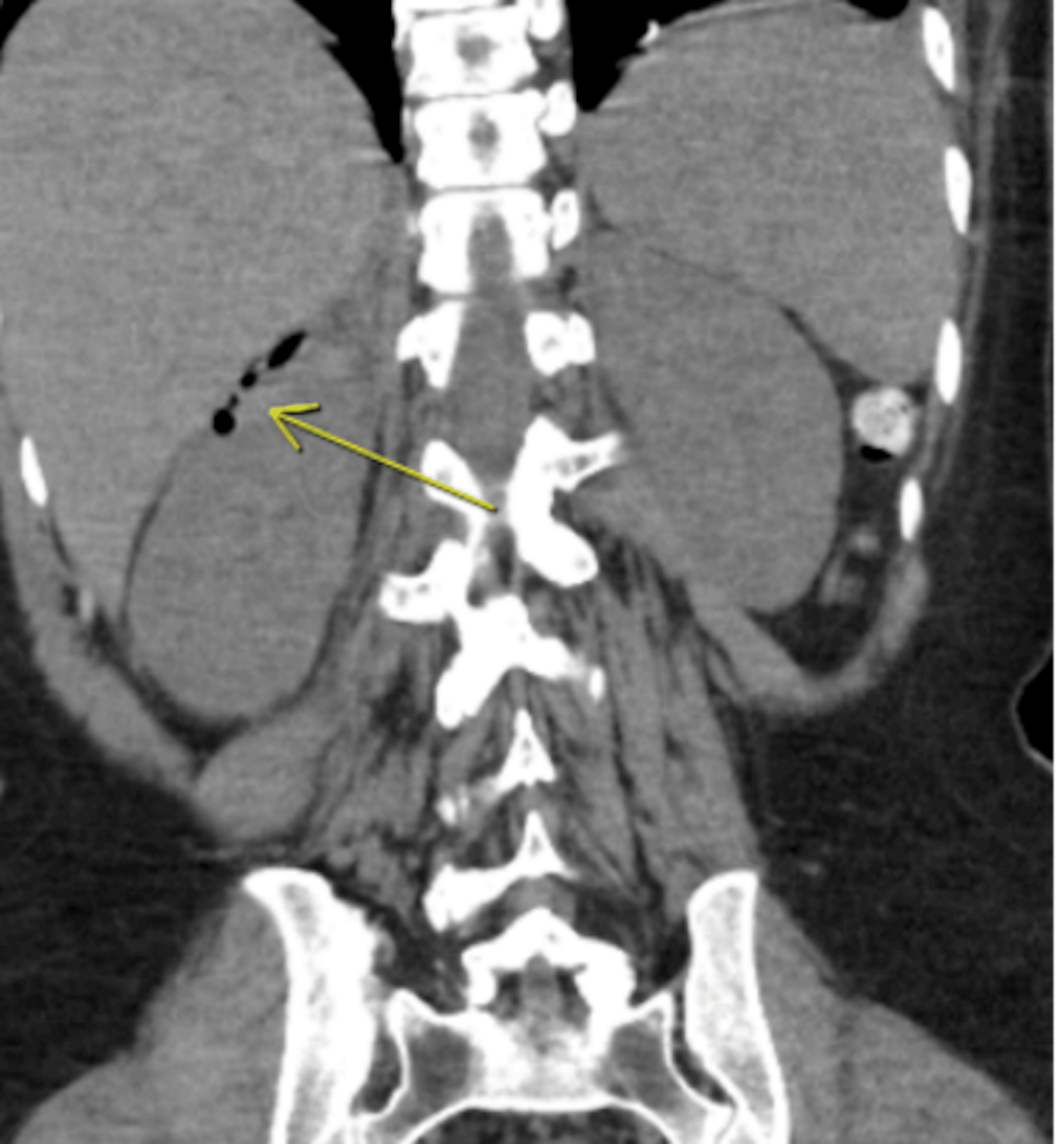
CT abdomen/pelvis coronal view showing subcutaneous emphysema in the right perirenal space.
